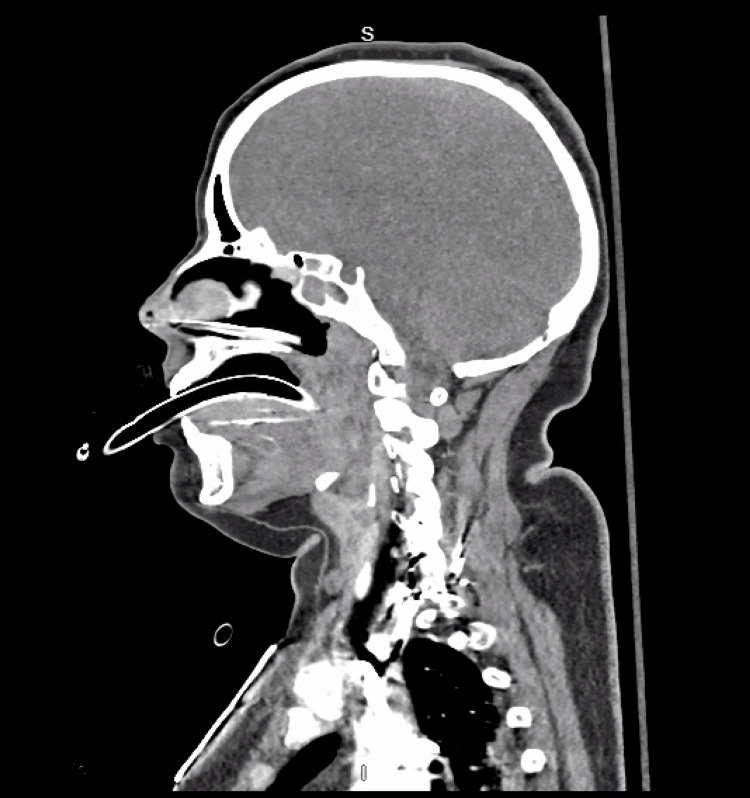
CT angiogram head and neck (sagital view) Preserved opacification of the extracranial carotid and vertebral arterial systems is observed, with absence of intracranial arterial filling. There is no visualization of the intracranial internal carotid arteries, circle of Willis, or distal cerebral branches. These findings are consistent with absent cerebral perfusion in the setting of markedly elevated intracranial pressure.
